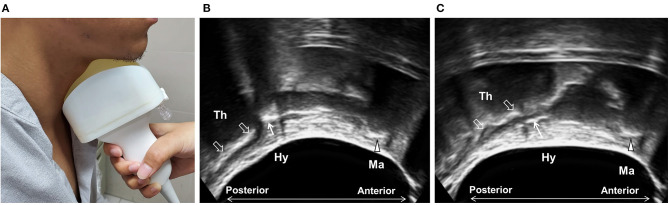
Ultrasonography using modified submental ultrasound transducer with water balloon. A water balloon was fixed in front of the transducer (A) to increase contact and to broaden the sonographic view, allowing for the simultaneous observation of the mandible (arrow head, Ma), the hyoid bone (arrow, Hy), and the thyroid cartilage (void arrows, Th) at rest (B) and during swallowing (C).
